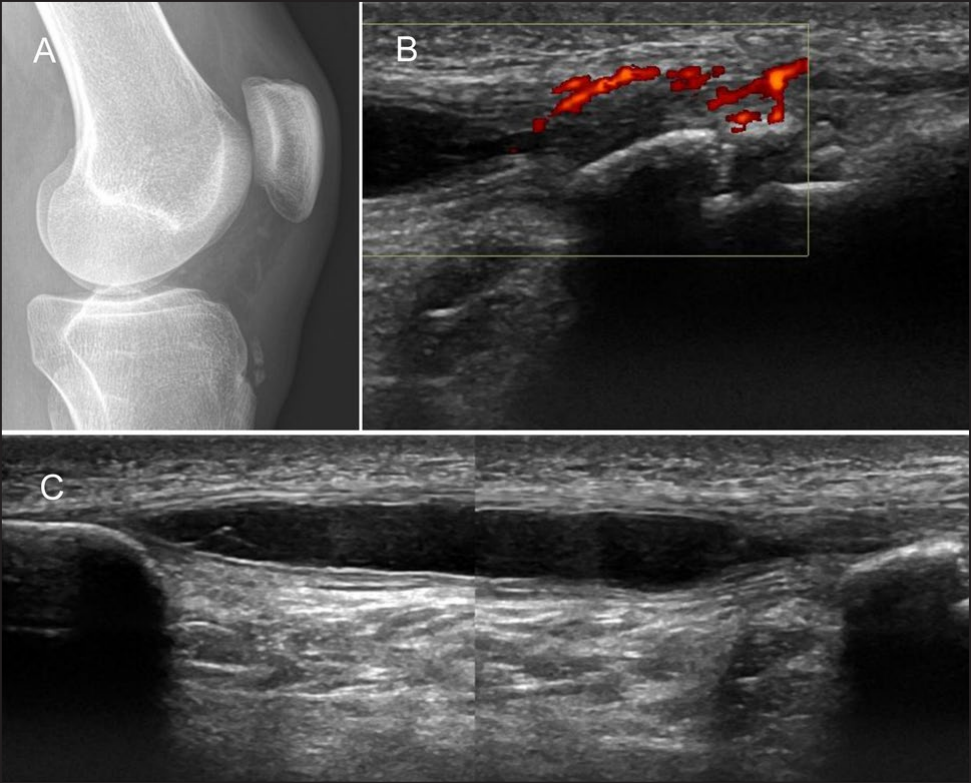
(A) Lateral X-ray of the knee; (B & C) High frequency ultrasound of the patellar tendon, sagittal plane.
